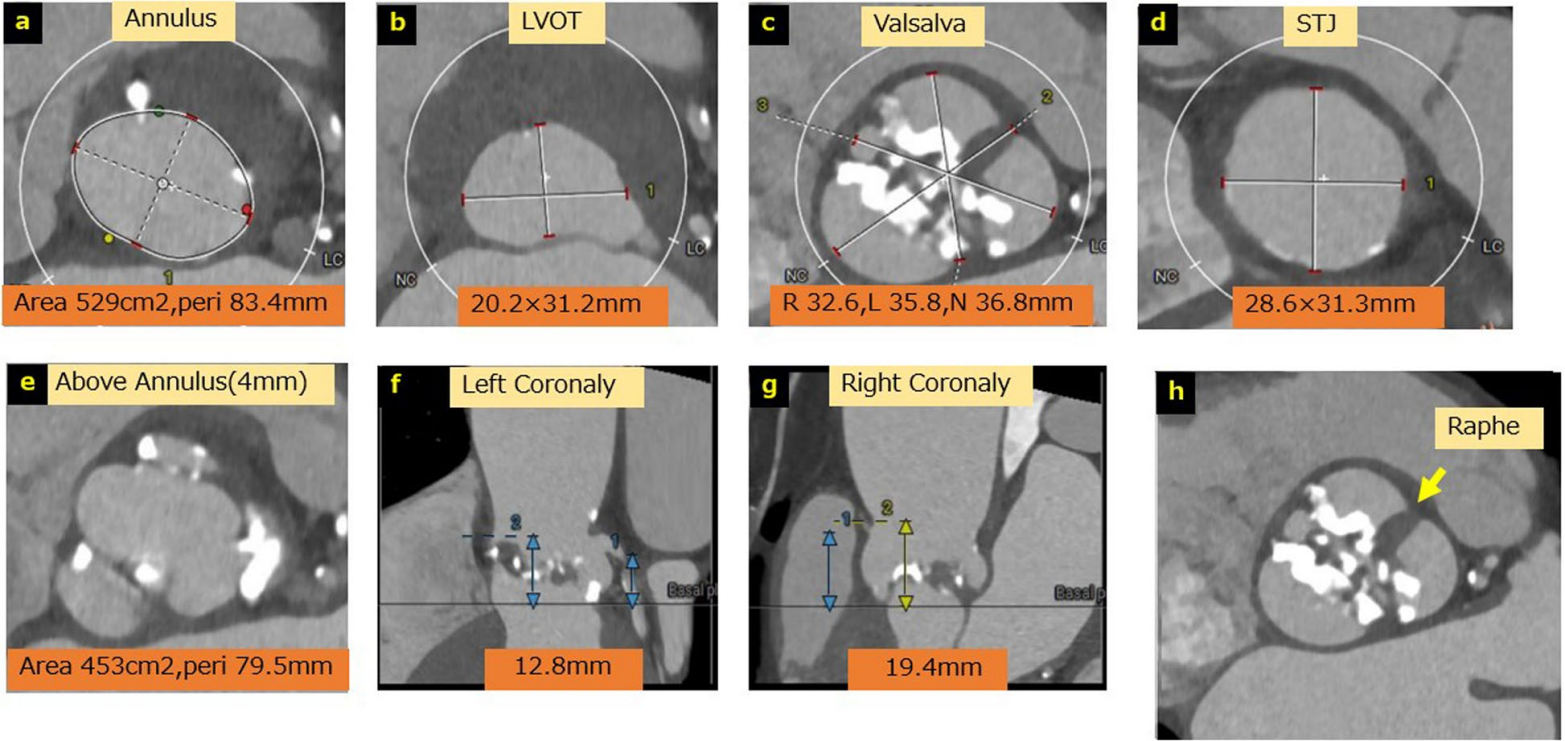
Preoperative contrast-enhanced computed tomography image of transcatheter aortic valve replacement. a Annulus; b left ventricular outflow tract; c Valsalva sinus; d sinotubular junction; e area 4 mm above the annulus; f left coronary height; g right coronary height; h: bicuspid valve, a type 1 bicuspid aortic valve with a R-L raphe is identified. *LVOT* left ventricular outflow tract, *STJ* sinotubular junction
